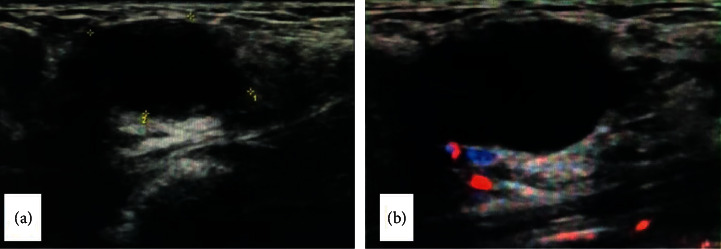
Ultrasonic examination. (a): hypoechoic nodule of the breast with a clear boundary; (b): no obvious blood flow signal in CDFI.
